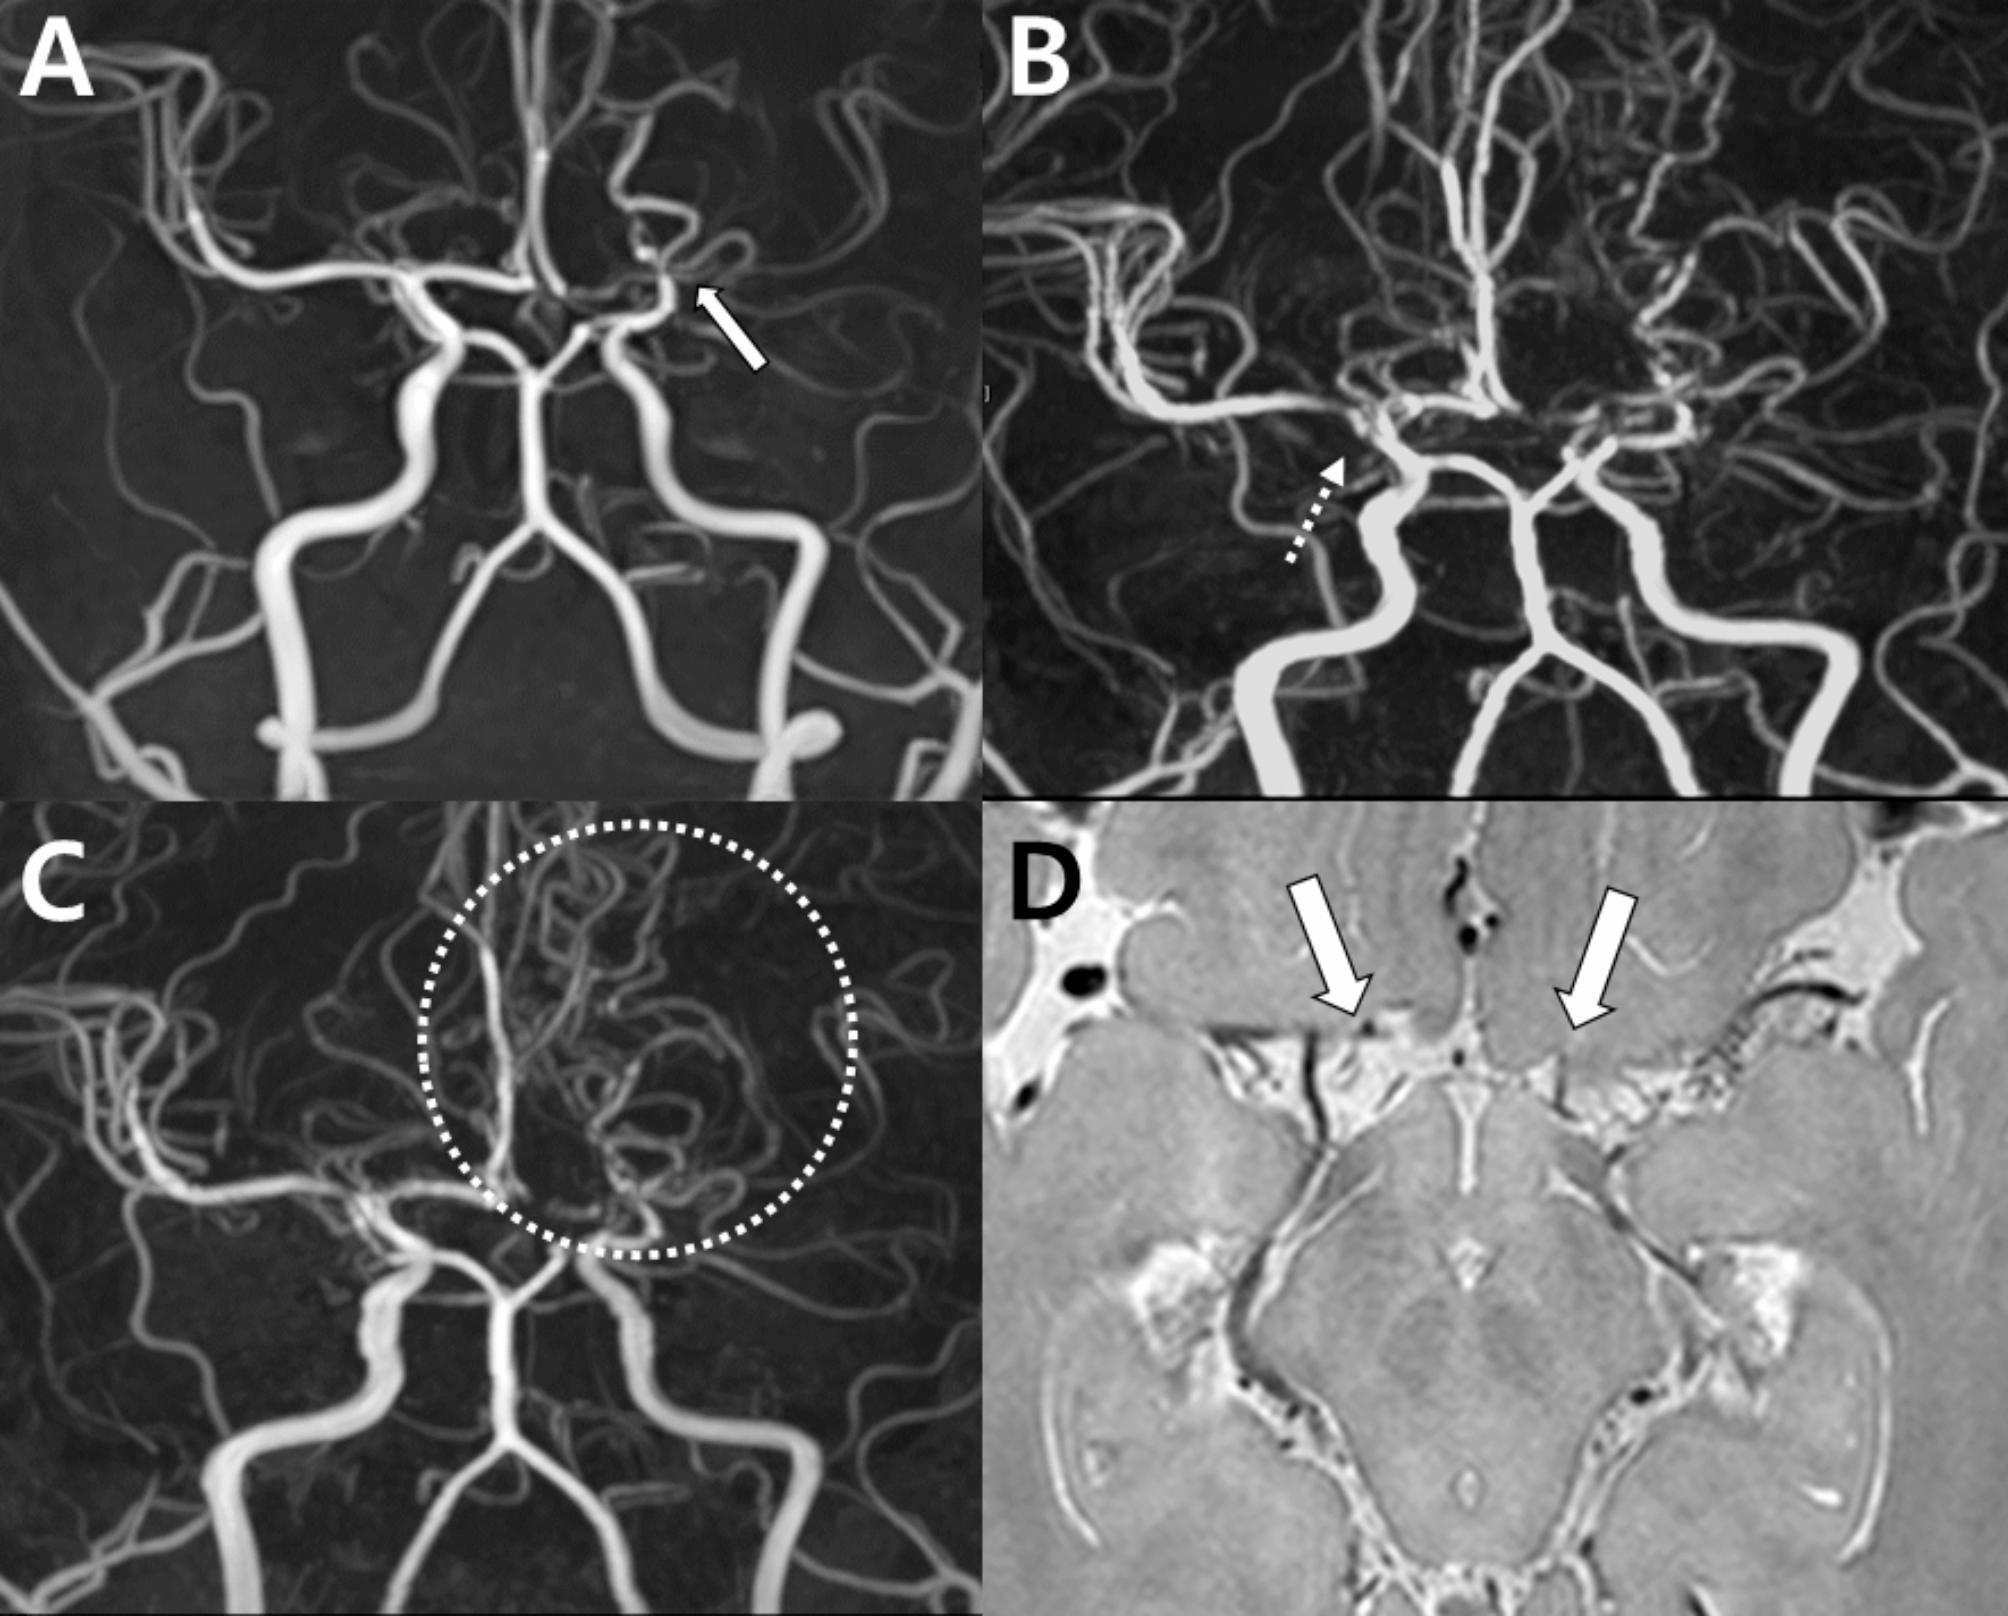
Mimickers of reversible cerebral vasoconstriction syndrome: unilateral moyamoya disease with rapid progression to bilateral disease (case 11). (A-C) Serial MRA angiographic imaging in a 30-year-old postpartum patient with recurrent transient ischemic attacks, right arm paralysis, and mild headache. (A) Initial imaging on the first day of onset shows isolated left distal ICA occlusion (arrow). (B) Subsequent imaging at 8 days after onset revealed rapid progression to right distal ICA stenosis (dotted arrow) along with left distal multifocal stenosis and a string-of-beads pattern. (C) At 1 year, no reversal of the bilateral distal ICA steno-occlusion or the vasoconstriction in the left distal arteries with formation of basal collateral vessels (dotted circle) were observed. (D) High-resolution vessel wall MRI showed significant obliteration of the ICAs (arrows), suggesting moyamoya disease. These cumulative observations led to the final diagnosis of moyamoya disease
